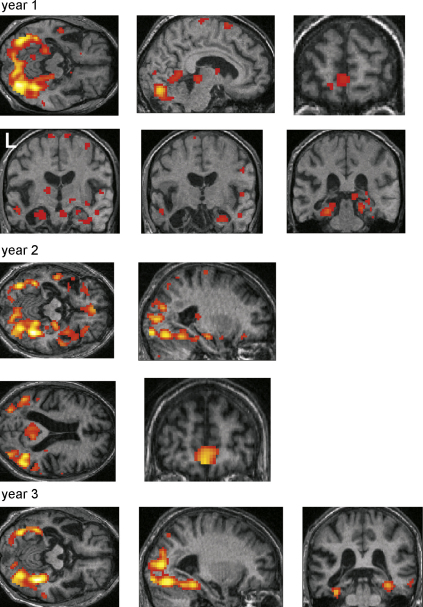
The brain networks associated with autobiographical memory retrieval in patient AM. Brain areas more active during autobiographical memory retrieval compared with the control task are shown for each year. Functional images are shown on a selection of relevant axial, sagittal and coronal sections from the patient's structural MRI scan contemporaneous with the functional images for that year. See Table 4 for full details of activation locations. Activations are shown at a threshold of *p* < 0.005 uncorrected. L: left side of the brain.
